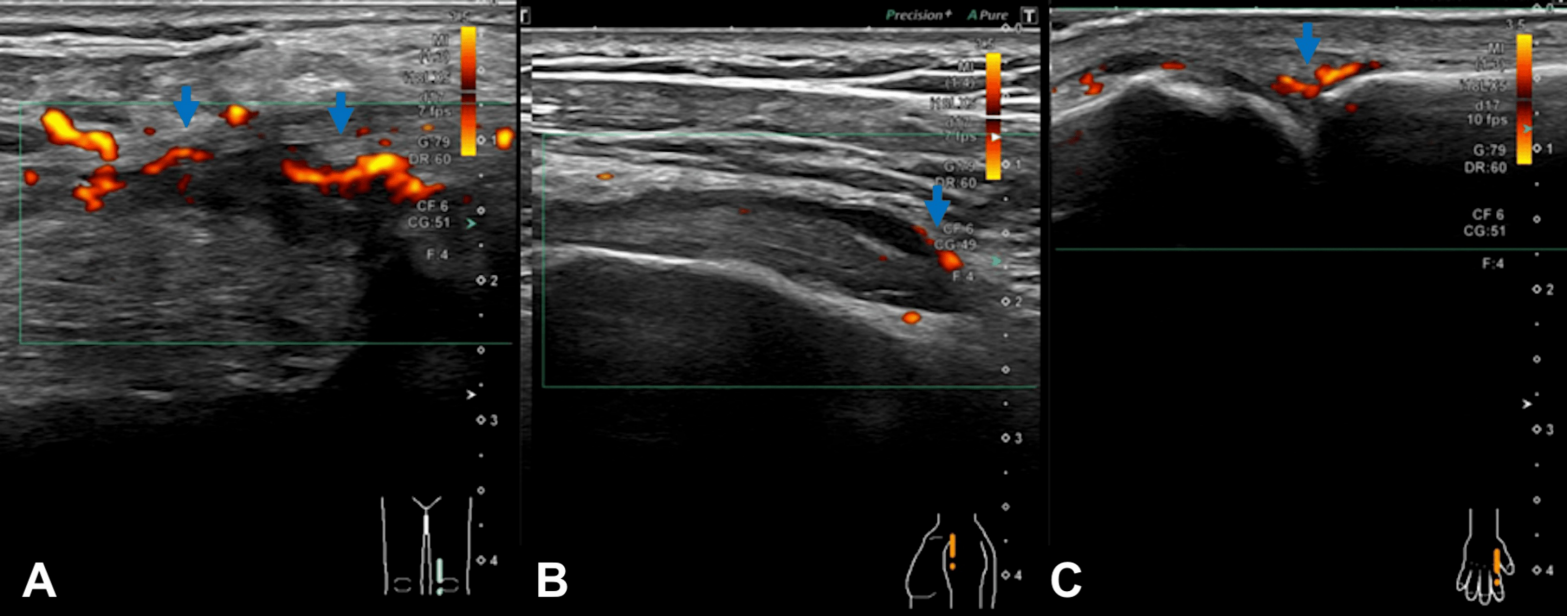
Ultrasonography of the (A) left knee, (B) shoulder, and (C) metacarpophalangeal joints demonstrating synovitis with high-power Doppler signals (blue arrows), a representative finding of the patient's polyarthritis.
